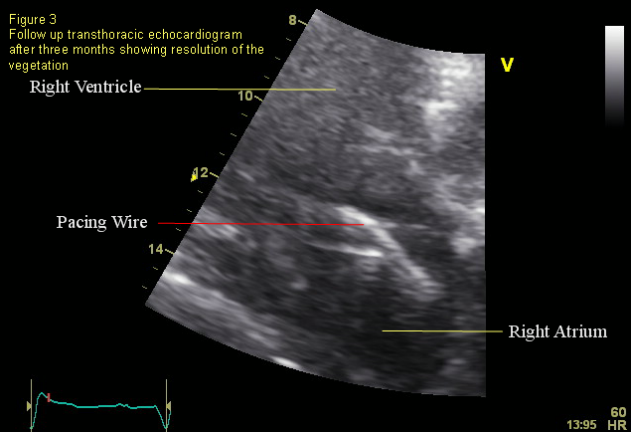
Labelled transthoracic image.
